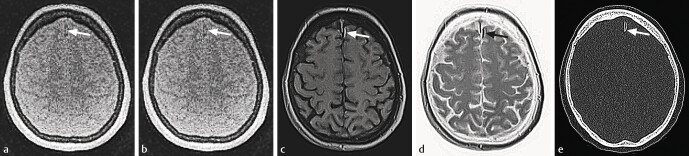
Comparison of ZTE vs. VIBE vs. CT for detection of calcification.
